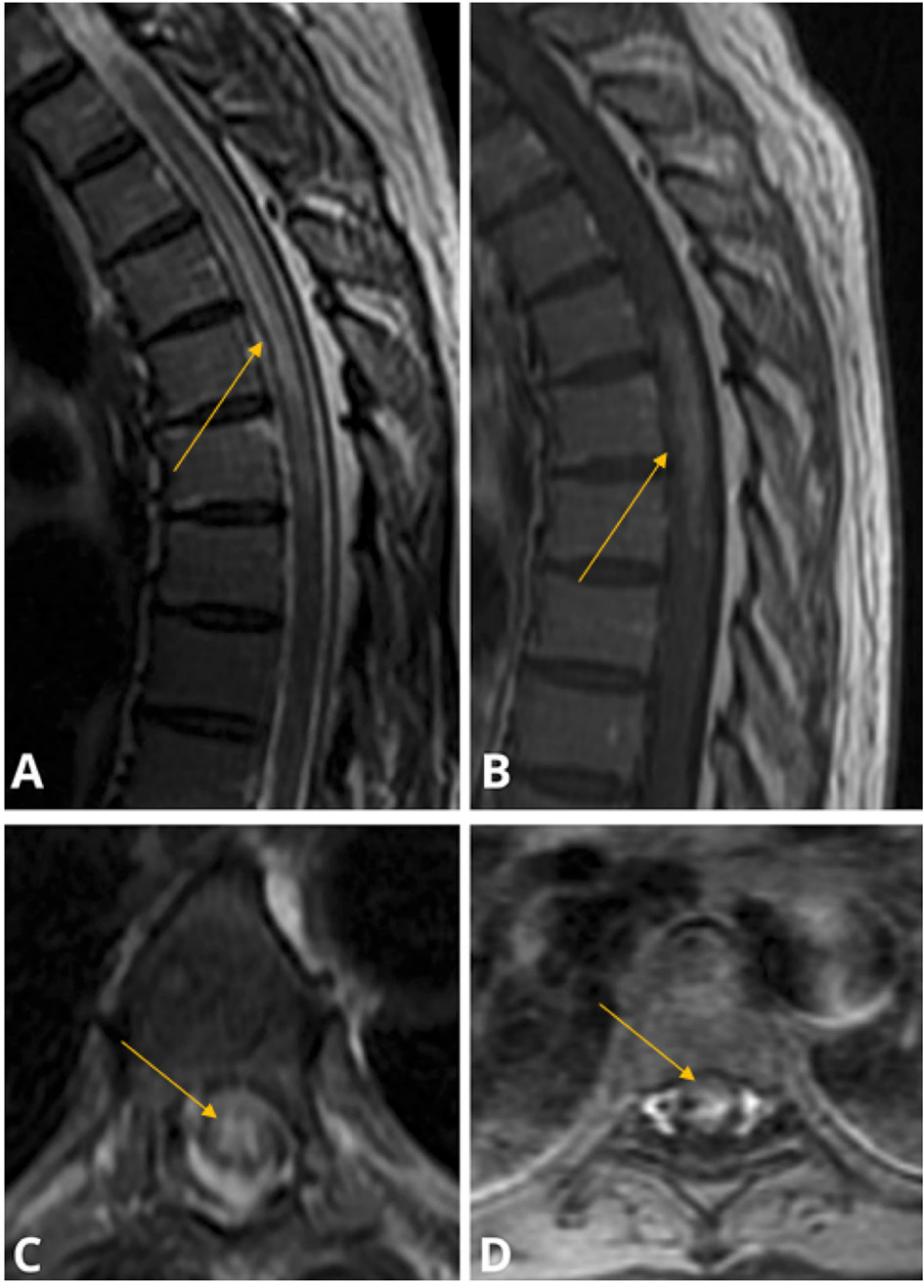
Case 3: Sagittal (A) and Axial (C) MRI T2 STIR demonstrating central gray-matter predominant hyperintensity spanning the T2–T9 vertebrae; Sagittal (B) and Axial (D) MRI T1 with gadolinium study demonstrating centrally enhancement spanning T5–T7 levels. The brain, cervical and lumbar cord were spared and thus not pictured.
